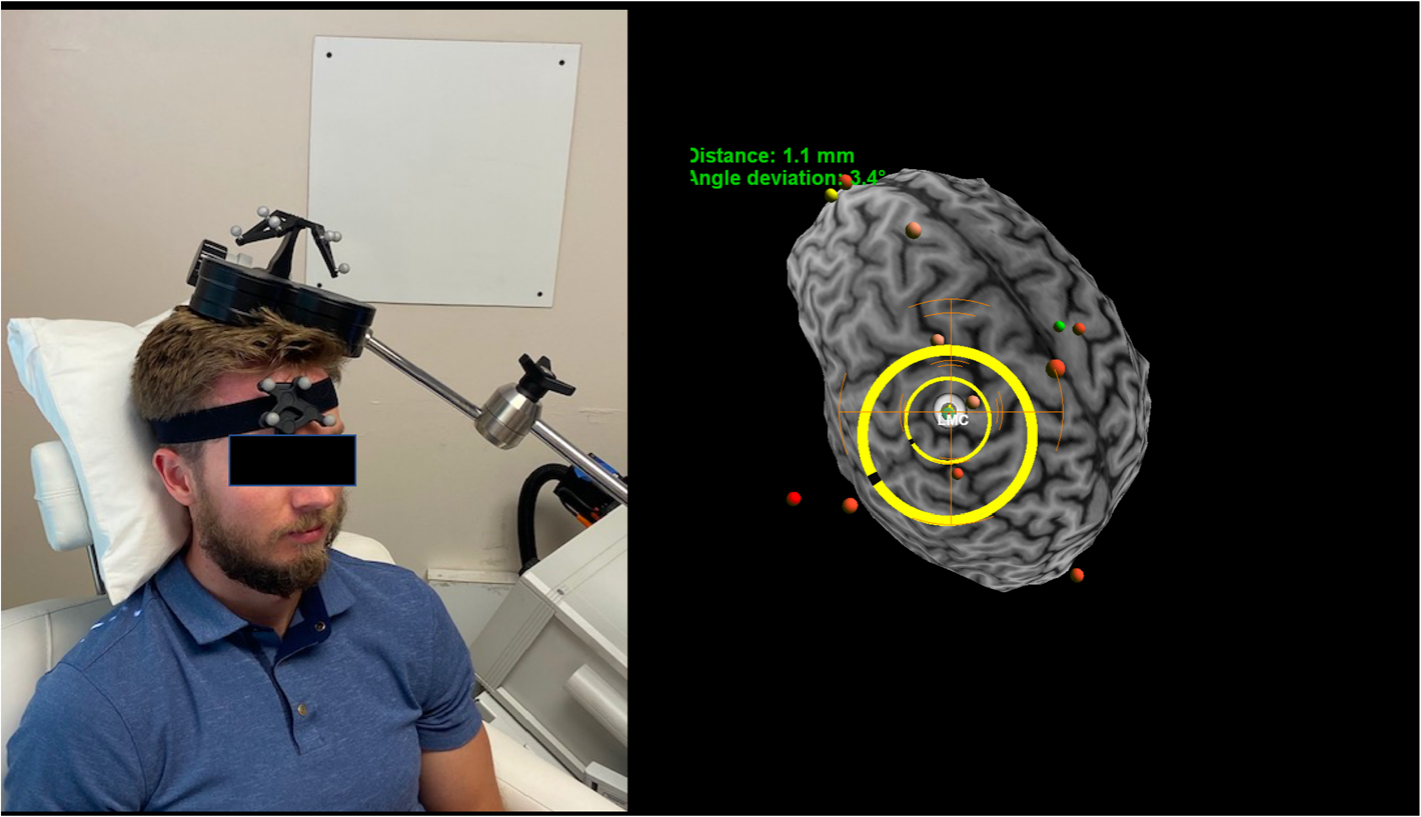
Magnetic resonance imaging neuronavigation guided transcranial magnetic stimulation at the left primary motor cortex
